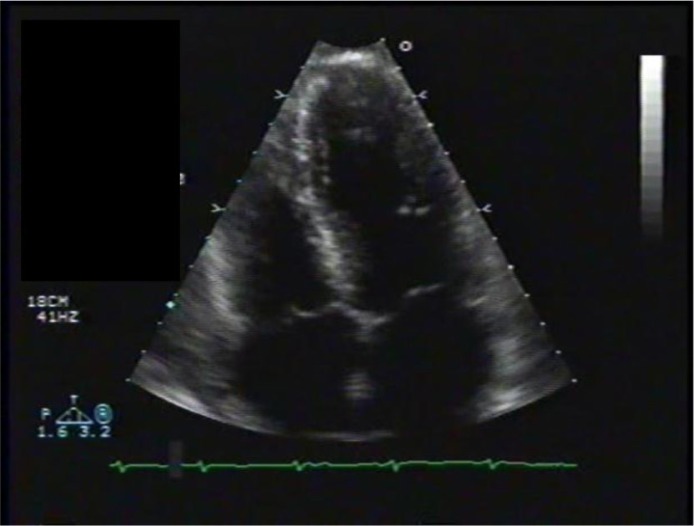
Echocardiogram, post-systolic. Note that there is not much difference between the post-systolic and the pre-systolic images suggesting some stunning of the myocardium.
